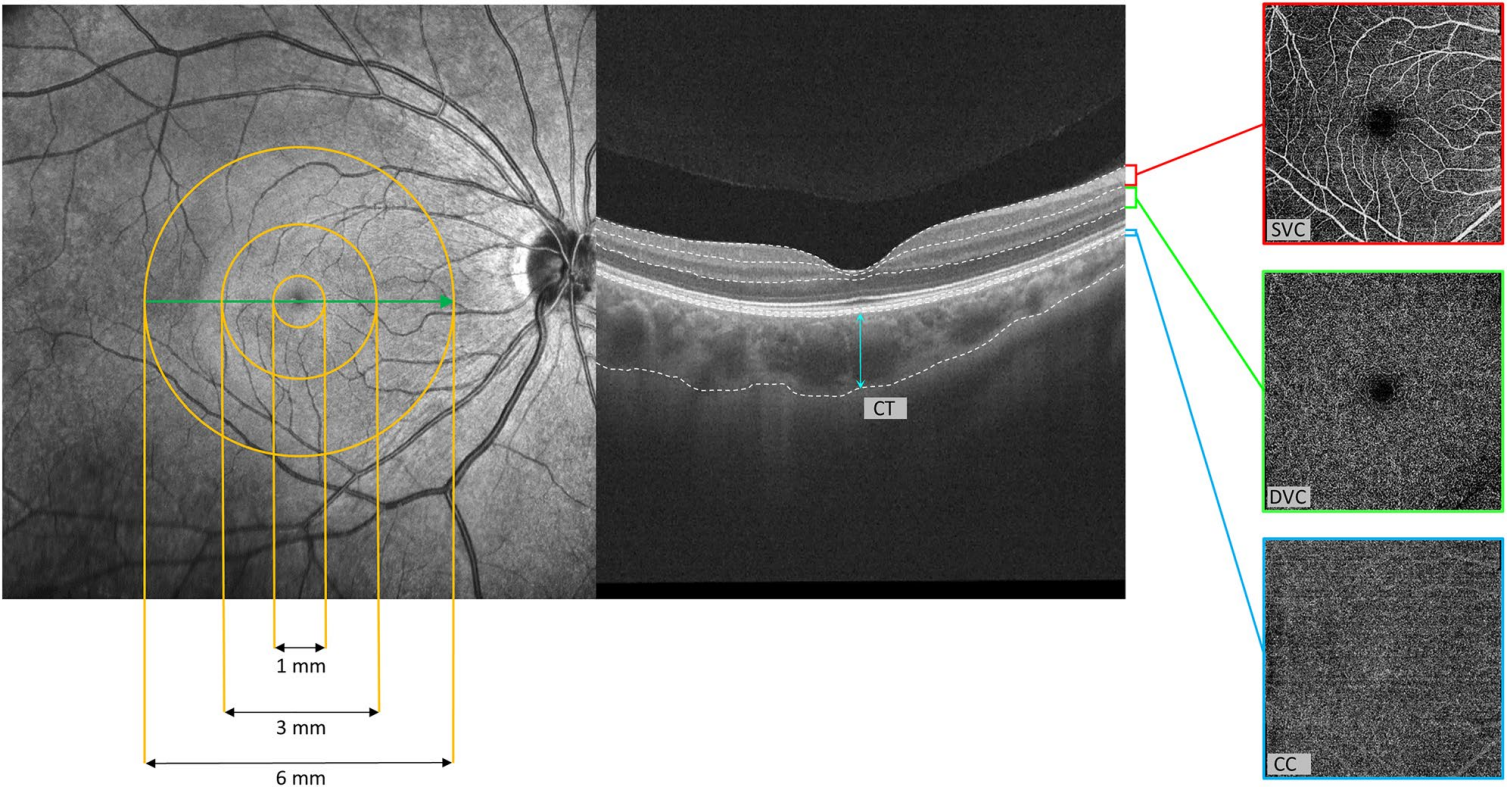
Segmentation of the macular subregions, structure, and microvasculature. *SVC* superficial vascular complex, *DVC* deep vascular complex, *CC* choriocapillaris, *CT* choroidal thickness.
